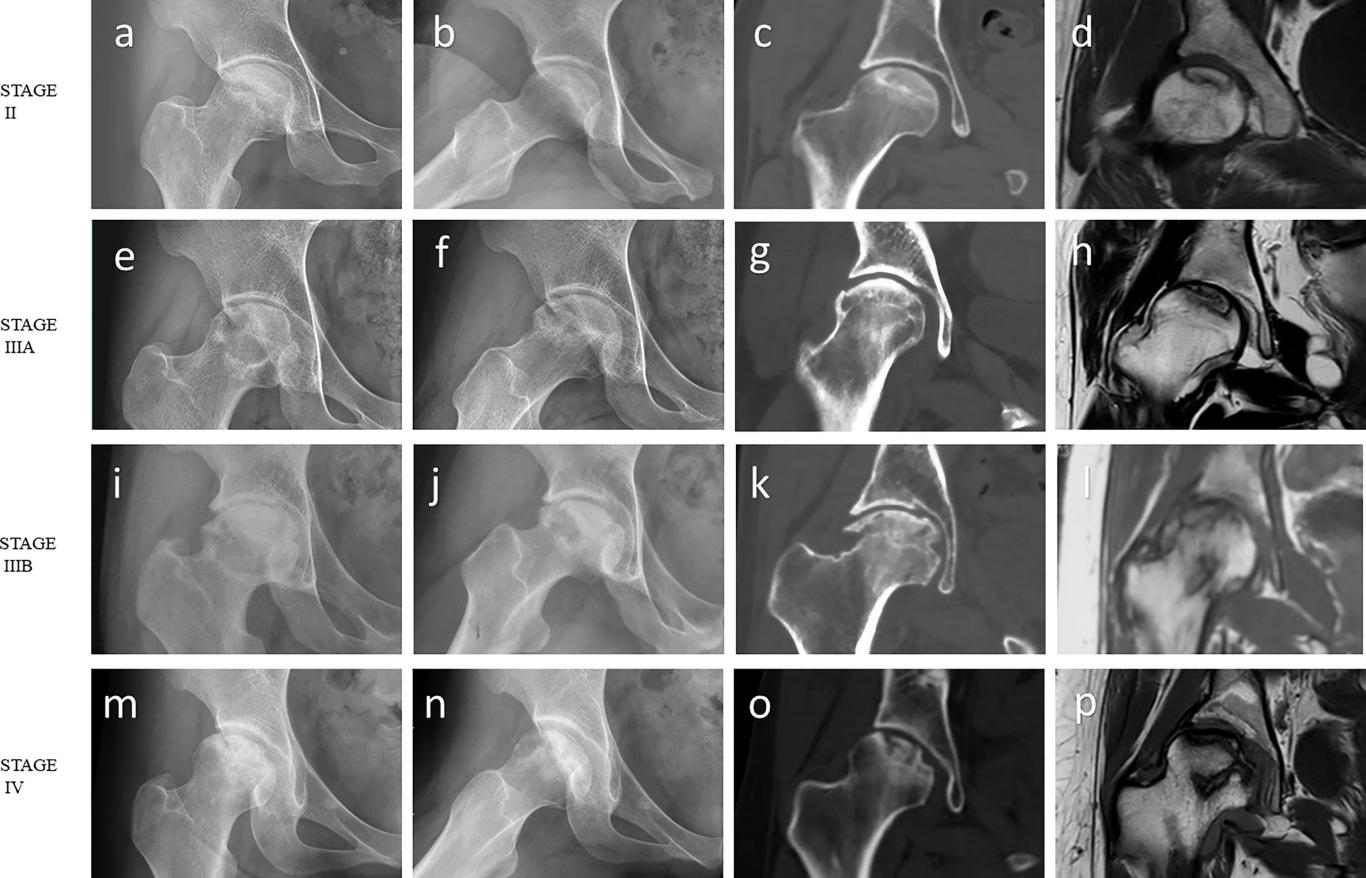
Imaging features of ONFH. (A-D) ARCO stage II: The radiograph showed ﻿focal osteoporosis and osteosclerosis in the femoral head. However, there were no signs of collapse, and the femoral head remained spherical on the coronal CT image and T1-weighted MR image. (E-H) ARCO stage IIIA: Subchondral fracture and ﻿flattening of the femoral head was observed on the radiograph or coronal CT image. Femoral head depression was less than 2 mm. (I-L) ARCO stage IIIB: Femoral head depression was more than 2 mm. (M-P) ARCO stage IV: Osteoarthritis of the hip joint with joint space narrowing was detected on the radiograph. Coronal CT image and T1-weighted MR image showed advanced degenerative alterations and entire joint destruction. Note: (A, E, I, M) ﻿Frontal radiograph; (B, F, J, N) Frog-leg lateral radiograph; (C, G, K, O) Coronal CT image; (D, H, L, P) Coronal T1-weighted MR image.
